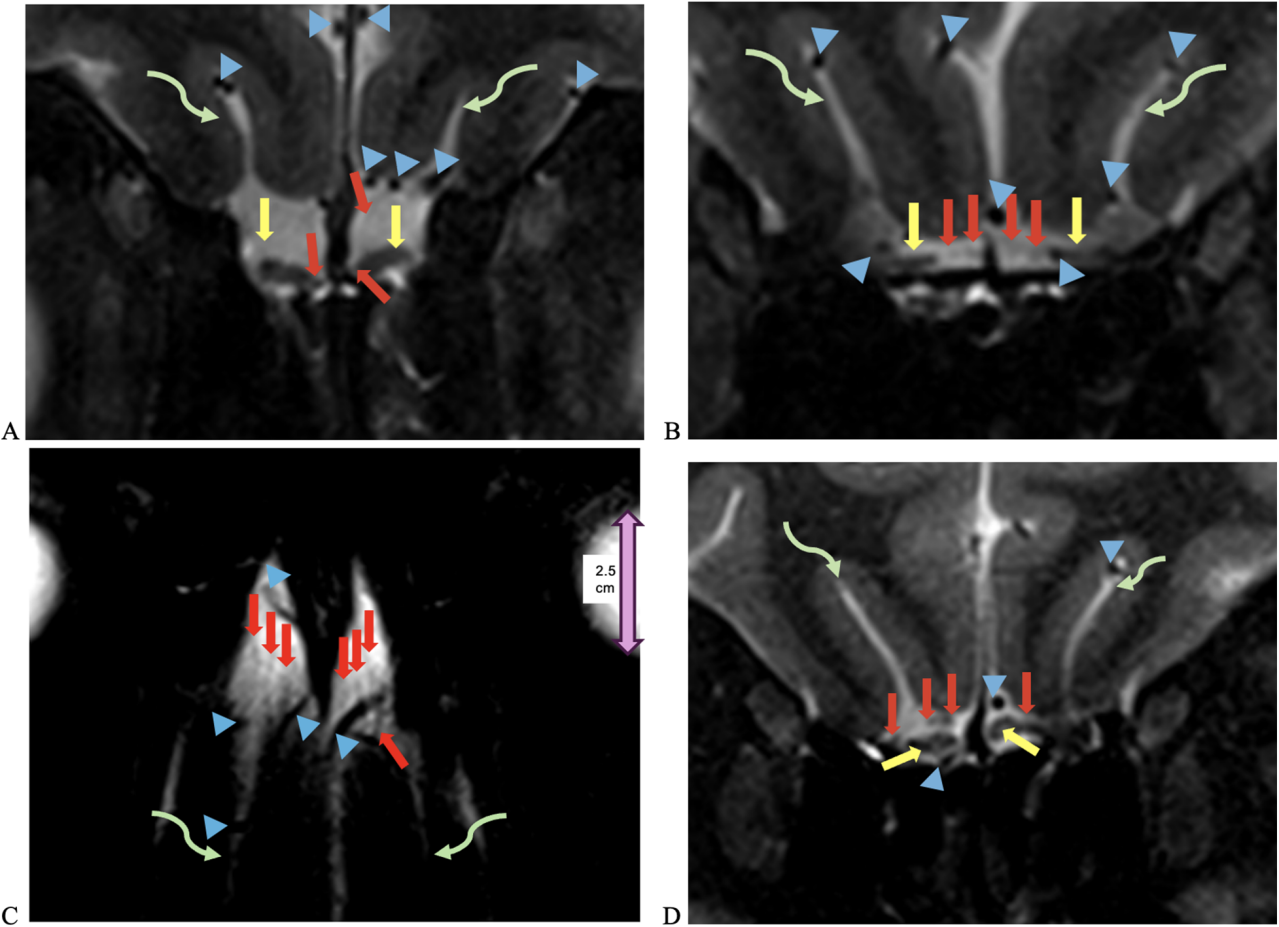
UHF 7-T MRI T2-weighted (A,B) coronal and (C) axial scans of a 38-year-old female healthy control. In (C), the orbital globe, pink dual arrow, measures 2.5 cm anterior–posterior for scale. (D) UHF 7-T MR T2-weighted coronal images of a 33- year-old female healthy control. Yellow arrows point to olfactory bulbs in (A,D) and olfactory tracts, red arrows point to the NT, blue arrowheads point to vessels with flow voids, and curved green arrows point to the olfactory sulcus. The depth of the olfactory sulcus in the coronal plane measures between 6.5 and 7.5 mm.
